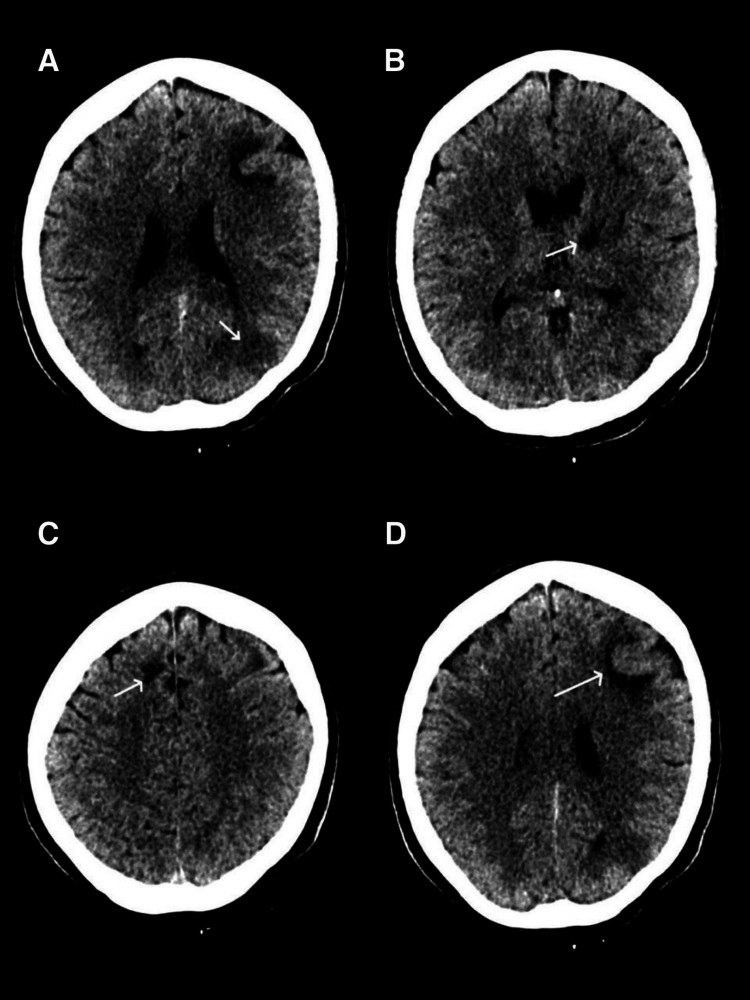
Non-contrast CT of the brain (A) Hypodense area in the left parietal lobe (white arrow), consistent with an acute infarct. (B) Chronic hypodensity in the left ganglio-capsular region (white arrow). (C, D) Irregular hypodensities in the bilateral frontal lobes (white arrows).
